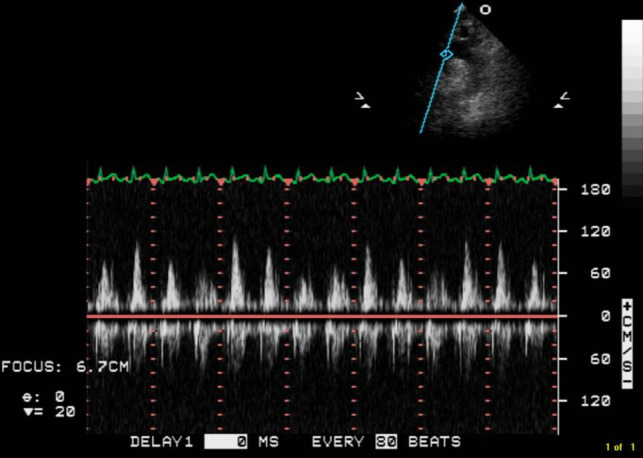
Transthoracic echocardiogram (TTE) in suprasternal view demonstrating exaggerated fall in systemic arterial flow velocities with inspiration—consistent with pulsus paradoxus
